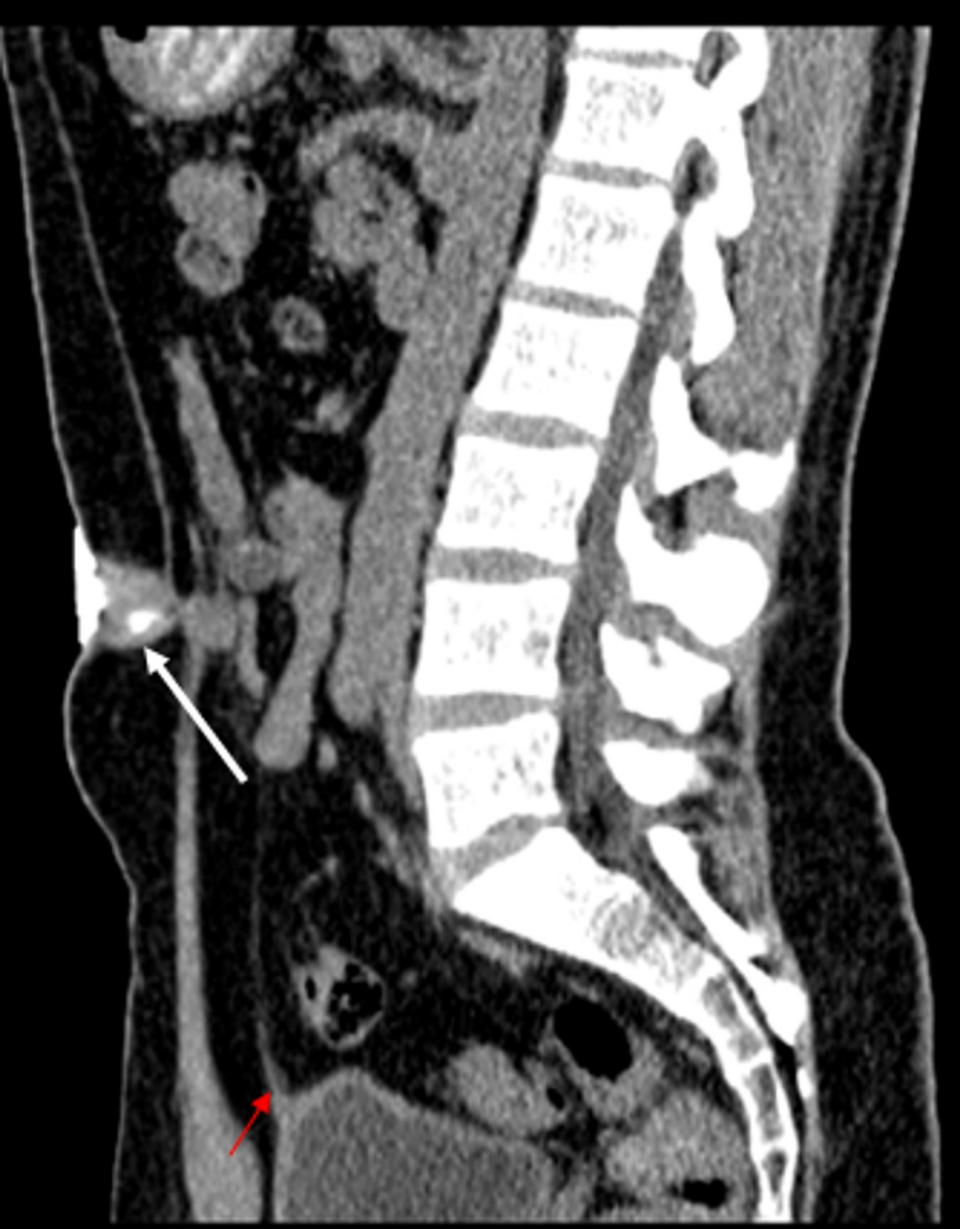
Midsagittal CT scan image following umbilical contrast instillation shows the partially filled tubular collection (White arrow) extending from the umbilicus to the median umbilical ligament (Red arrow). No evidence of communication with the bladder lumen is seen.
